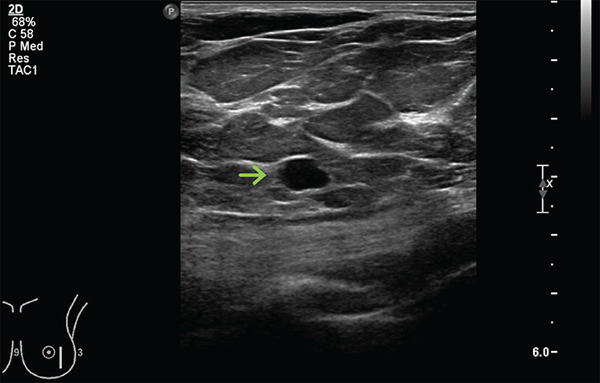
Ultrasound of the left breast in antiradial projection demonstrates a 9‐mm circumscribed, anechoic simple cyst with posterior acoustic enhancement at the 4 o′clock position (green arrow), corresponding to the mammographic finding in Figure 3. BI‐RADS 2 (benign).
